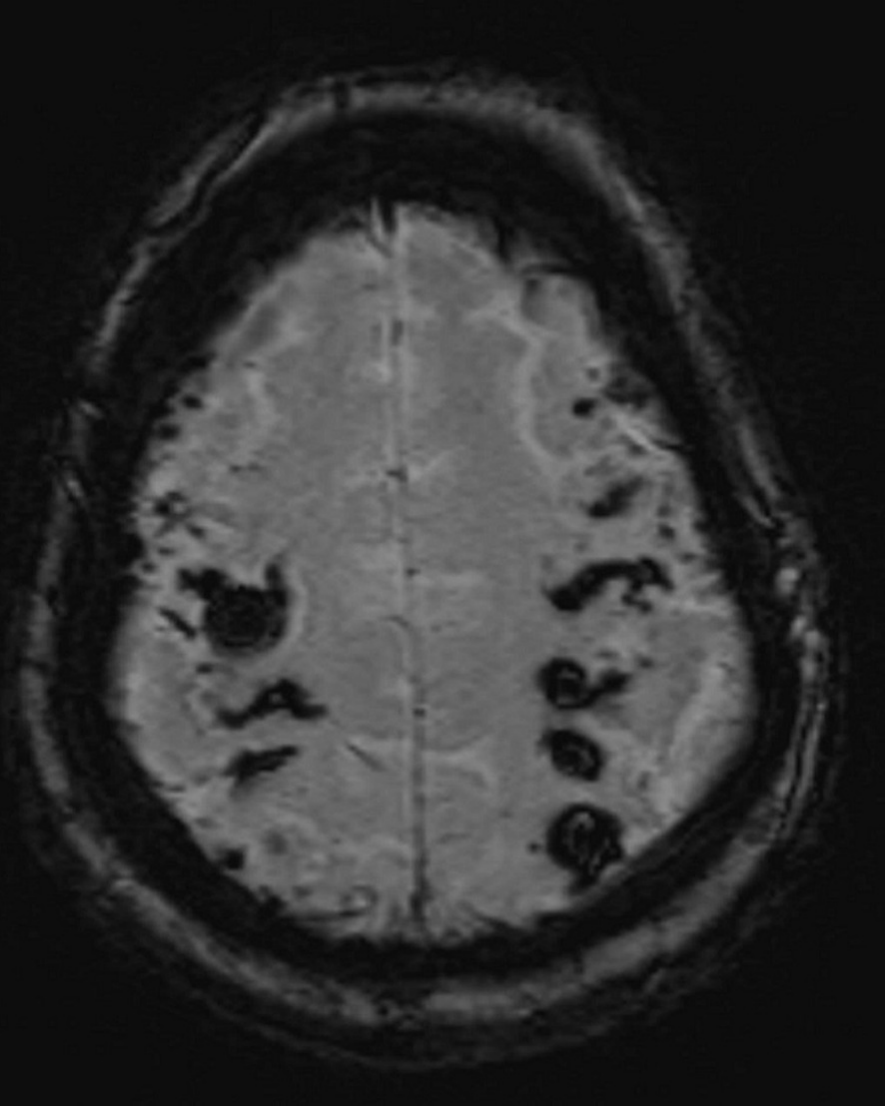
Maximum intensity projection susceptibility weighted image sequence (mIP SWI) MRI demonstrating haemosiderin deposition in the cerebral sulci.
